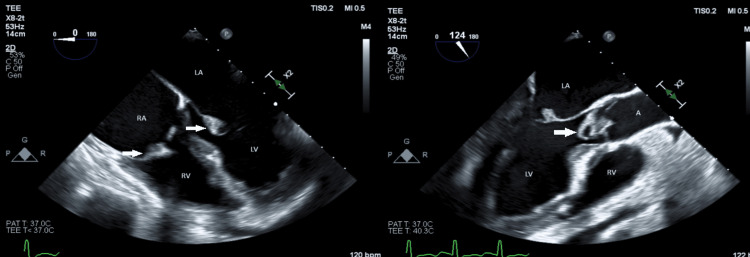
Transesophageal echocardiography showing vegetations (white arrows) in tricuspid valve, mitral valve, and aortic valve RA: right atrium, LA: left atrium, RV: right ventricle, LV: left ventricle, A: aorta
